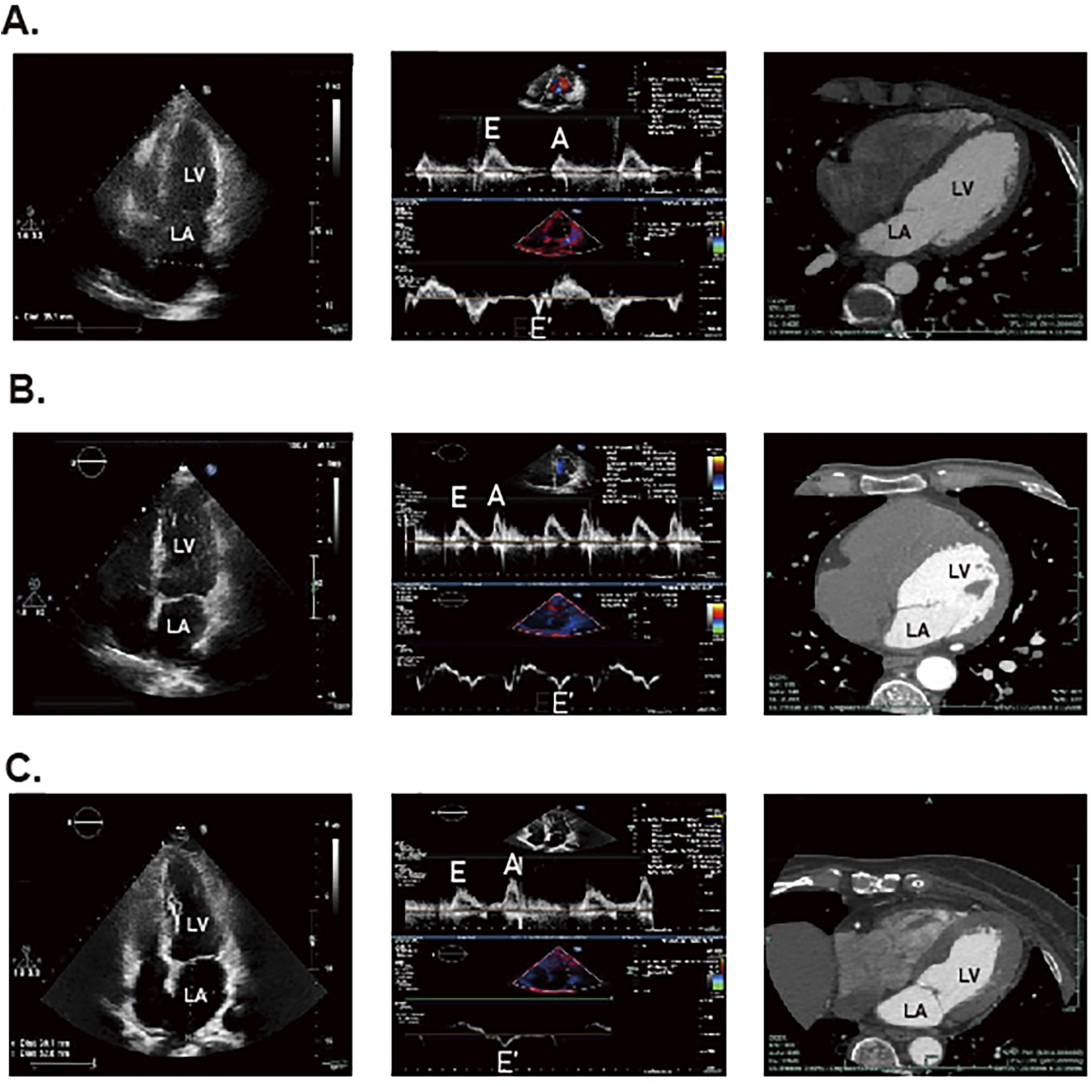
The STE and CT results for DCM patients, DM patients and healthy individuals. The panel (A) represents the result of healthy individuals. The panel (B) represents the result of patients with DM. The (C) panel represents the result of patients with DCM.
